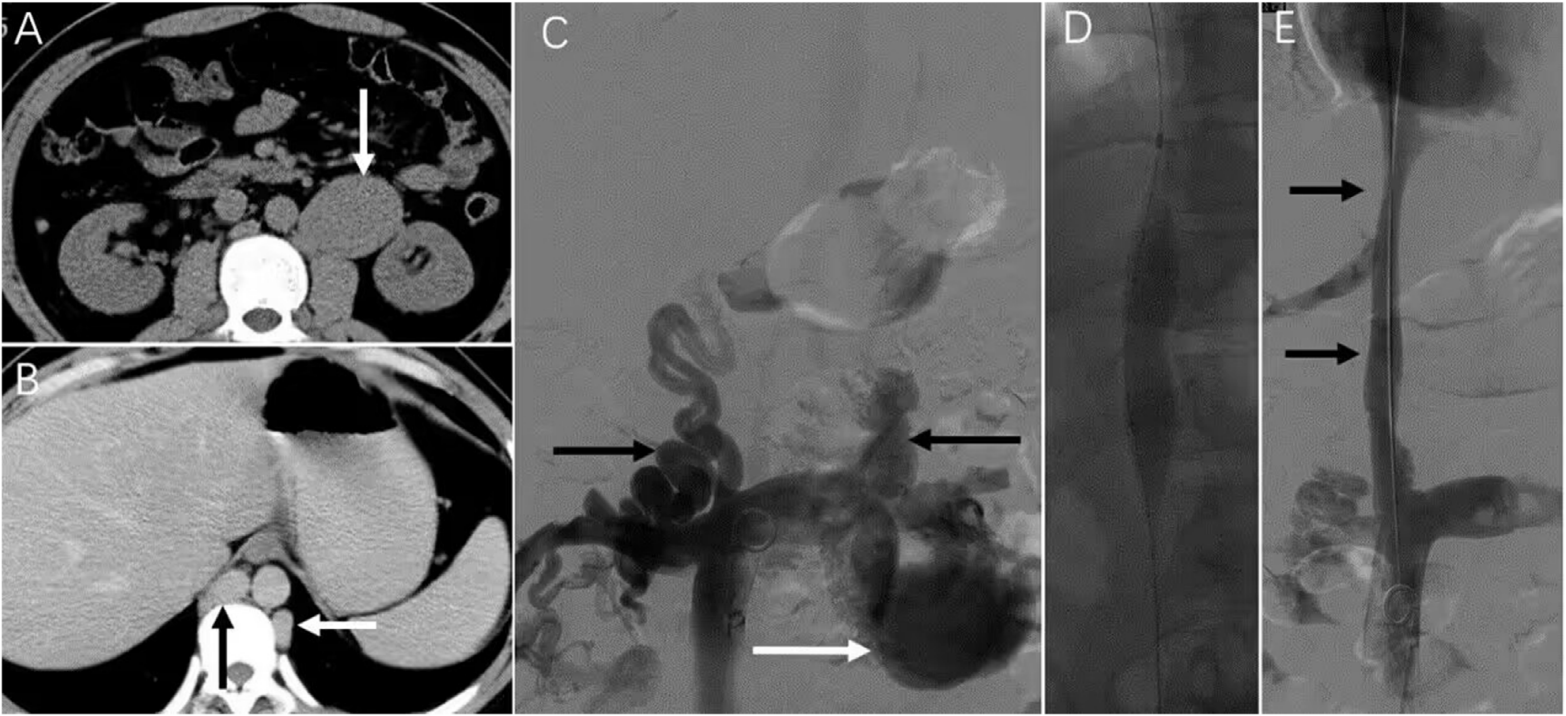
Serious complications that may be encountered in endovascular treatment. Note: The patient in case 3 presented with persistent abdominal distension for the past 6 months. (A) Upon a CT examination, a retroperitoneal tumor was suspected. (B) Further investigation through an enhanced CT scan revealed the presence of a left renal vein aneurysm and the absence of the IVC. The azygos and Hemiazygos vein displayed significant dilation (indicated by black and white arrowheads). (C) Vascular angiography was conducted, which confirmed the absence of the suprarenal IVC and the development of collateral circulation (indicated by a black arrowhead). A left renal vein aneurysm was also observed (white arrowhead). (D) Subsequently, dilation of the IVC was noted. (E) Following the vascular intervention, the IVC regained its normal patency (indicated by an arrow).
